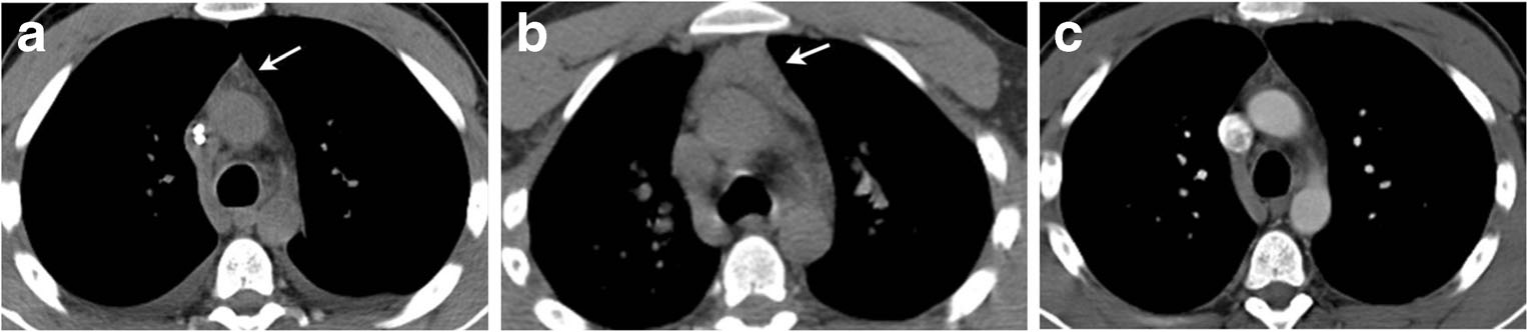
A 22-year-old male with relapsed HL shows thymic rebound after second-line chemotherapy. a Axial non-contrast CT image shows triangular low-attenuation anterior mediastinal soft tissue (*arrow*) suggestive of normal thymus. b Axial non-contrast CT image after 3 months of chemotherapy shows diffusely enlarged thymus (*arrow*). The thymic contour is maintained with smooth margins and absence of surrounding vascular invasion. c Axial non-contrast CT image 4 months after completion of chemotherapy shows resolution of thymic enlargement with similar appearance of thymus compared to pretreatment study
